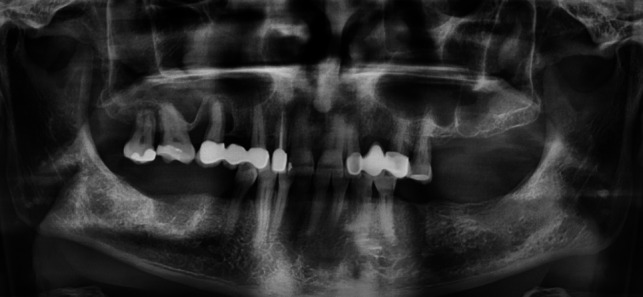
Panoramic radiograph before treatment by the OMS. The horizontal bone accretion is consistent with periodontitis. In addition, tooth 17 and 15 (according to FDI-scheme) show apical/periradicular radiolucency consistent with apical/periradicular periodontitis. Related to this is the radiodense basal right maxillary sinus as an expression of odontogenic sinusitis
